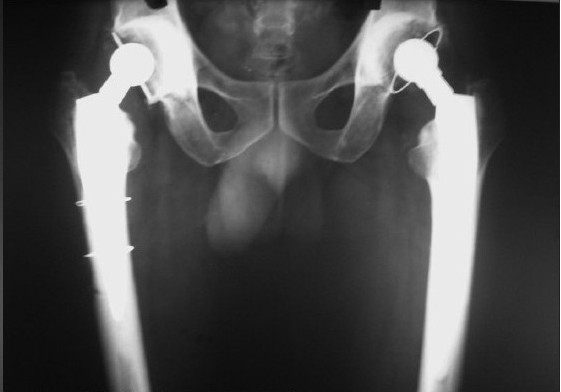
X-ray pelvis with both hips of the same patient showing minimal shortening and subsidence at 11 years follow up
